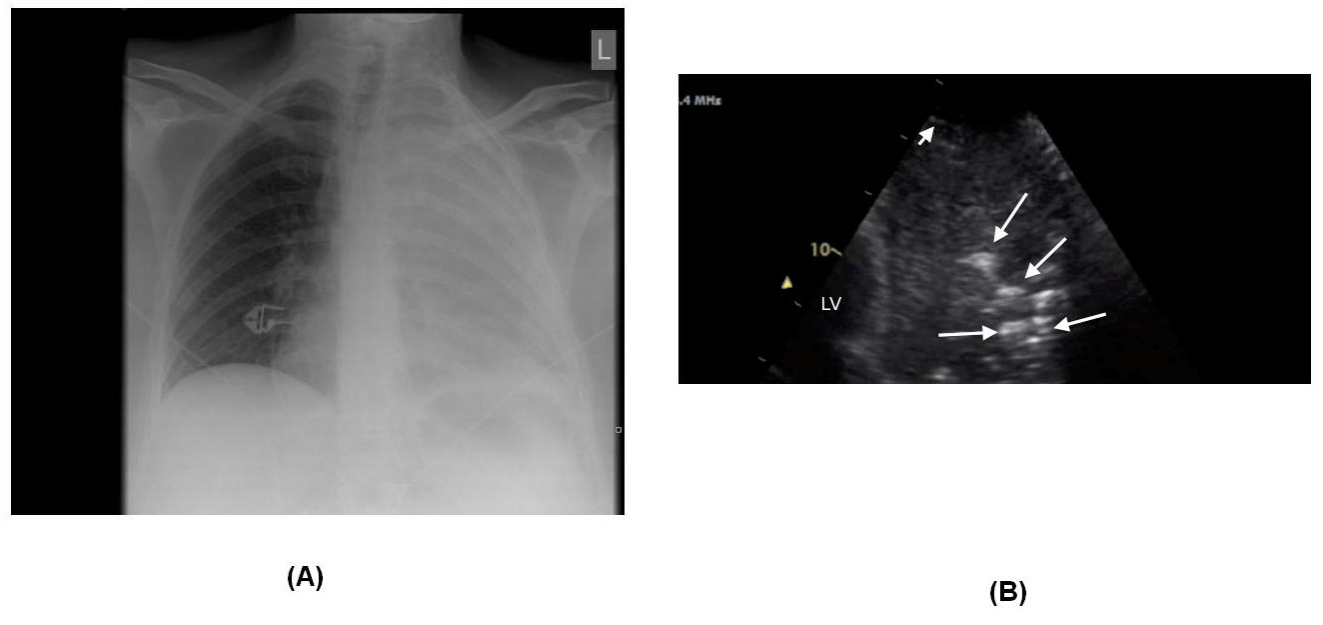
Chest X-ray and ultrasound of patient 1. (A) Chest X-ray showing complete opacification of the left lung. (B) Chest ultrasound showing pneumonia characterized by an irregular hypoechogenic area with air bronchograms and many hyperechogenic areas (long white arrows). The pleural line was hypoechogenic (short white arrow) as is frequently observed.
